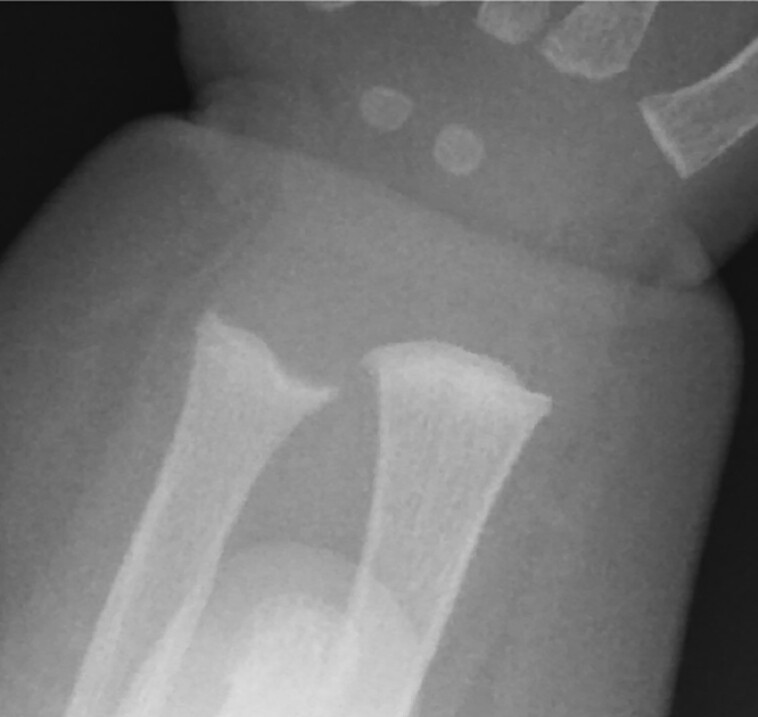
Radiograph of the left wrist, age 8.5 months.
